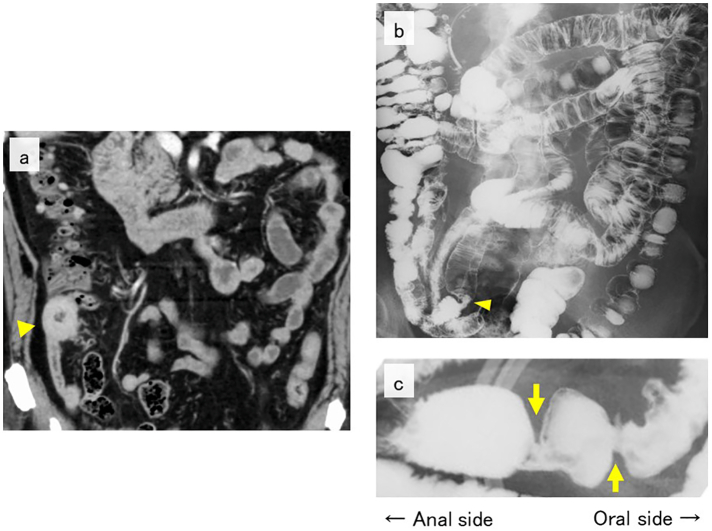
Radiological examinations. Contrast-enhanced CT shows circumferential wall thickening in the terminal ileum (a, arrowhead). Enterography shows a stenotic lesion 30–35 cm on the oral side from the terminal ileum (b, arrowhead), but no obstruction was observed in the entire gastrointestinal tract. Multiple stenotic lesions are shown (c, arrows); however, gastrografin could still pass through.
